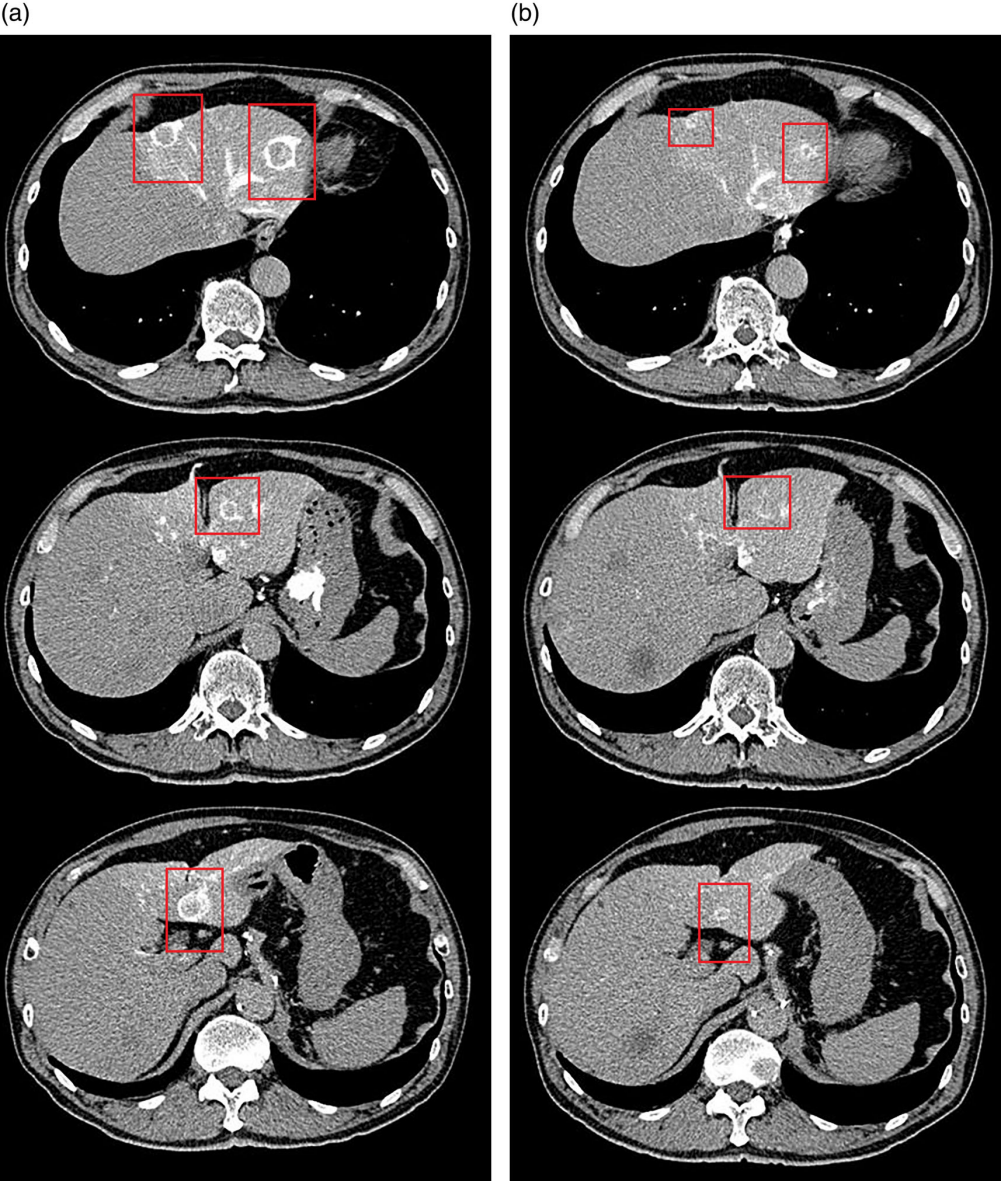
(a) Computed tomographic angiogram showing a high‐density area in the left lobe in computed tomography during left hepatic arteriography (CTLHA) in the second phase. (b) The second‐phase CTLHA demonstrated a necrotic cyst and tumor regression of the tumor in the left lobe after a 3‐month follow‐up treatment.
